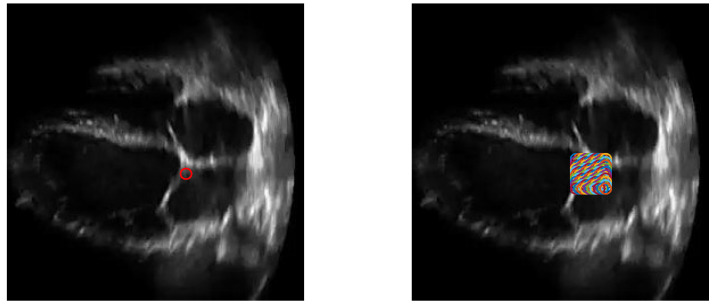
An ultrasound image with one initialization point (left) and 100 points (right).
